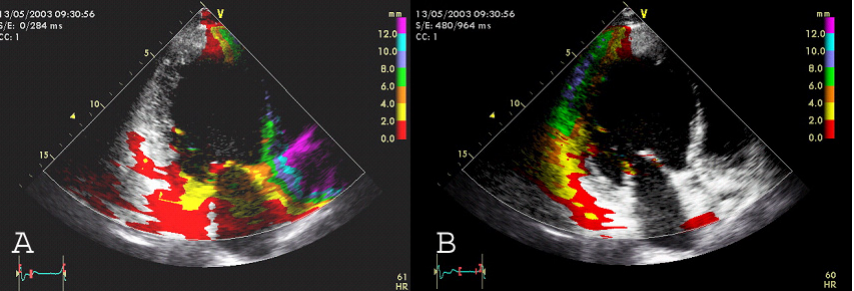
Delayed longitudinal contraction (=DLC) as a marker for asynchrony can be visualized by analysis of systolic and diastolic Tissue Tracking. Systolic tracking analyzes the systolic displacement i.e. tracking interval between the onset of QRS-complex and the end of the T-wave. Diastolic tracking can demonstrate DLC with colour coding (end of T until begin of R). Images from apical two chamber view A) Systolic Tracking: The inferior segments (=grey area) show DLC with no systolic motion B) Diastolic Tracking: The inferior segments (=colour coded area) show DLC with diastolic movement.
